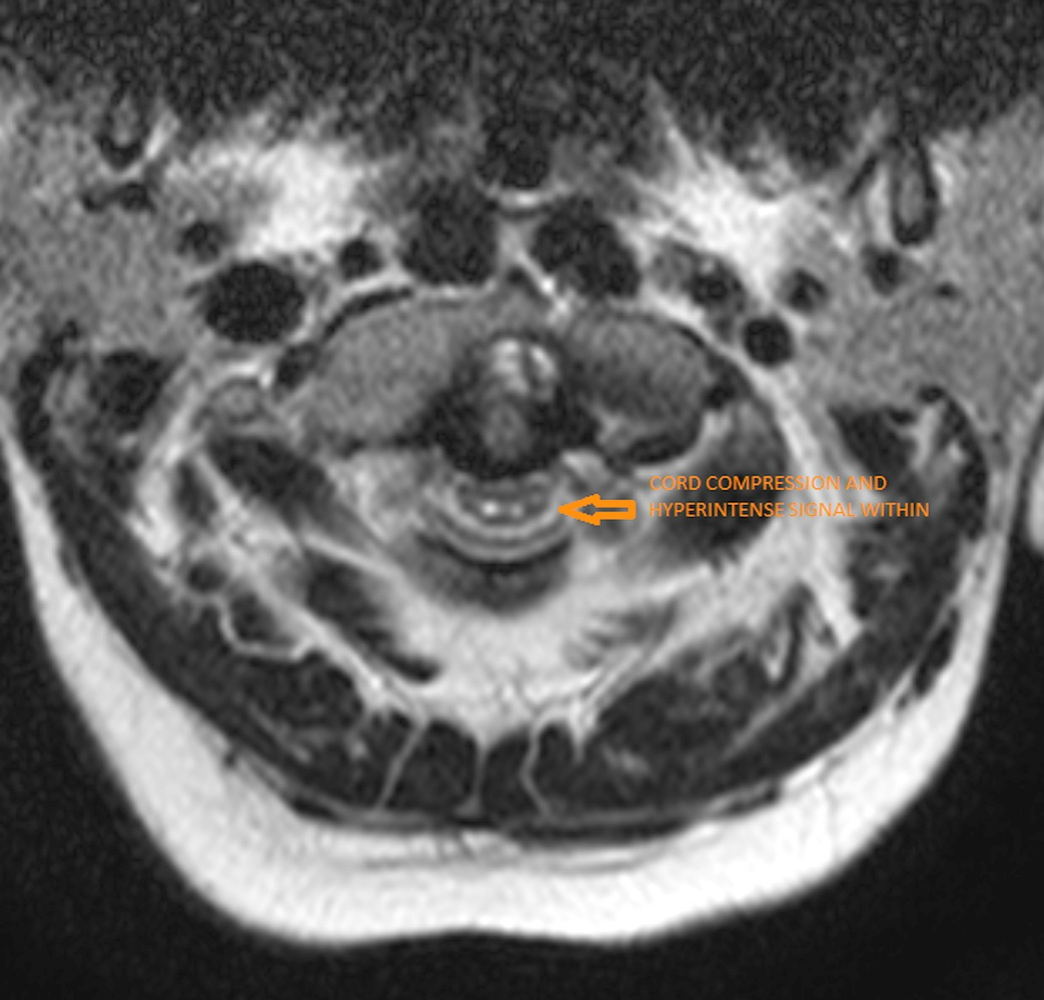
Axial T2-weighted magnetic resonance imaging showing compressive myelopathy
